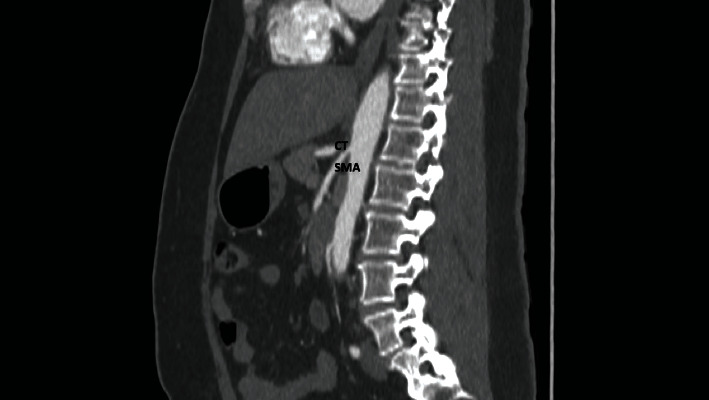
Follow-up CT scan at 8 months showing resolution of the thrombus in the superior mesenteric artery.
